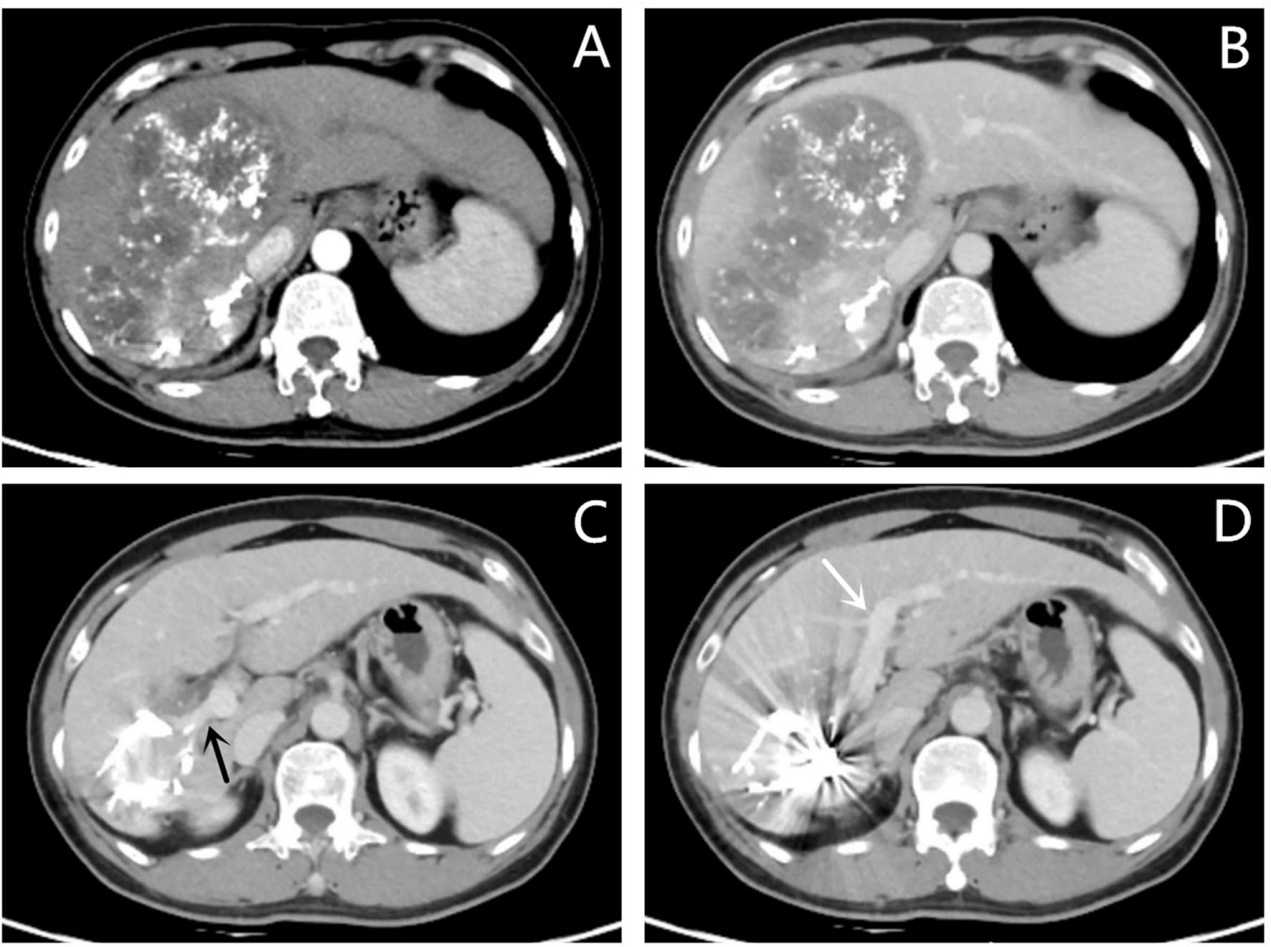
Images of follow-up contrast-enhanced CT of the same patient as in Figures 1, 2 after PVE and three sessions of transarterial chemoembolization. (A, B) Hepatic arterial phase (A) and portal vein phase (B) showing that the lesion is fully necrotic. (C) Images of the portal vein phase showing a clear initial part of the right portal vein (black arrow). (D) Images of the portal vein phase showing a clear left portal vein (white arrow). Compared with Figure 1, the left lobe of the liver proliferated significantly after PVE.
